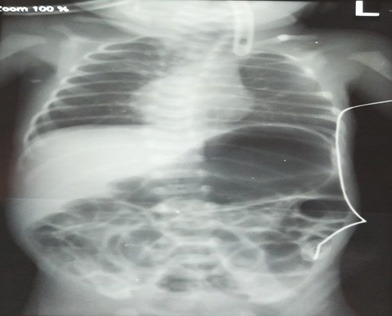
X-ray showing red rubber tube not passing beyond 10 cm and gastric distension indicating oesophageal atresia with tracheoesophageal fistula.
